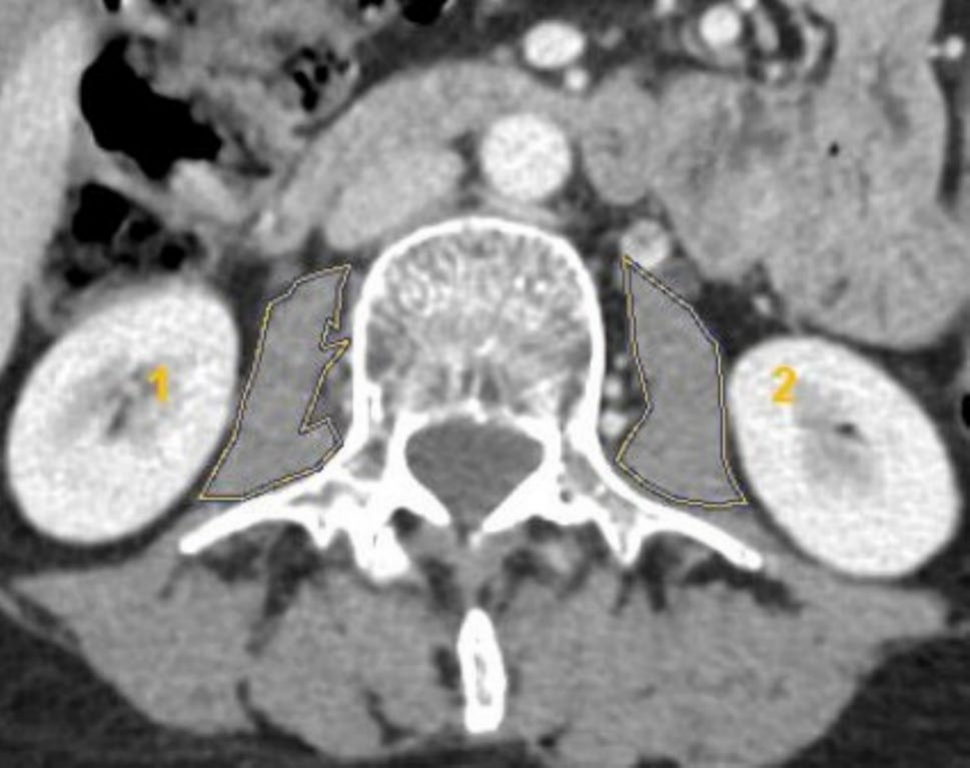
Measuring of psoas muscle areas on the axial plane CT at the level of L3 vertebra
